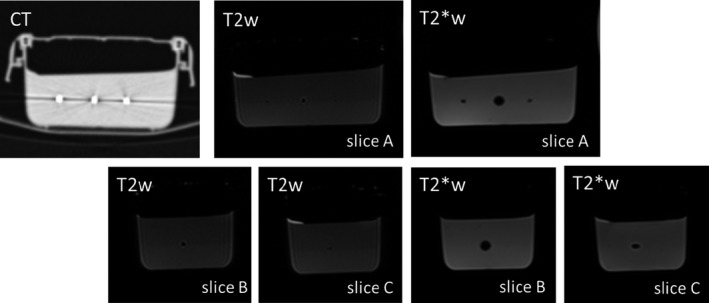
Gel test object images showing signal loss around marker positions, which is larger on T2*W images as expected. The signal loss is symmetric in relation to the true position of the marker. The level of signal loss associated with the markers varies, and is much larger for the central marker, irrespective of test object orientation. Ultrasound and CT images confirm there is no air gap or any imperfection at the markers. Image intensity differences within the gel in T2W images are due to the test object construction technique, in two layers; the second layer is built after the bottom layer has hardened sufficiently to hold the weight of the seeds.
